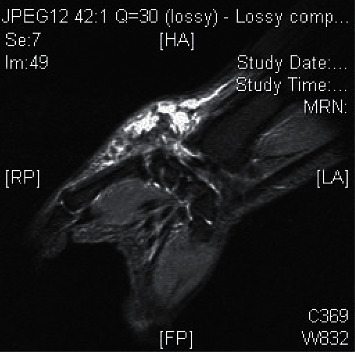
Magnetic resonance imaging (T2-weighted) of the left wrist.
